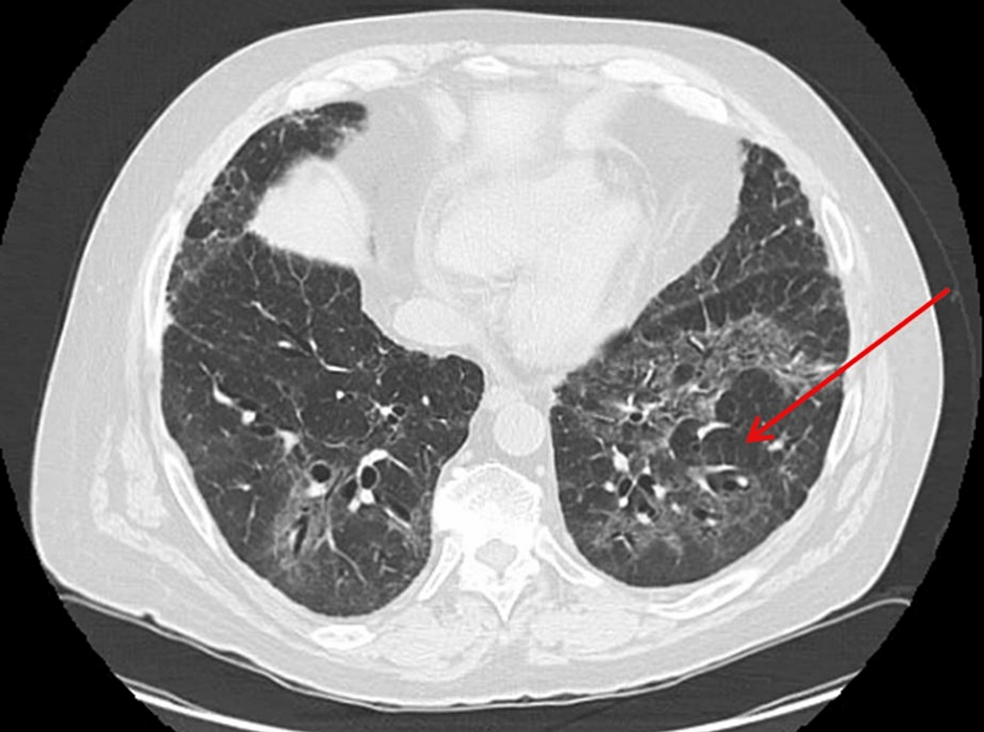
High-resolution CT findings two years after GPA diagnosis High-resolution CT of the chest two years after GPA diagnosis, on rituximab, shows bilateral ground-glass opacities and broncho-centric consolidation, with reversed halo sign (atoll sign) in the left lower lobe (red arrow). It also shows traction bronchiectasis and peripheral reticulation CT: computed tomography; GPA: granulomatosis with polyangiitis
